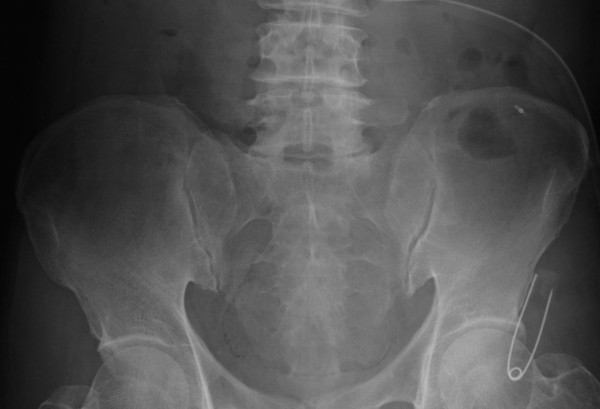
Plain radiograph of the abdomen showing a nonspecific gas pattern and a small amount of air in the true pelvis, in the shape of the bladder.
